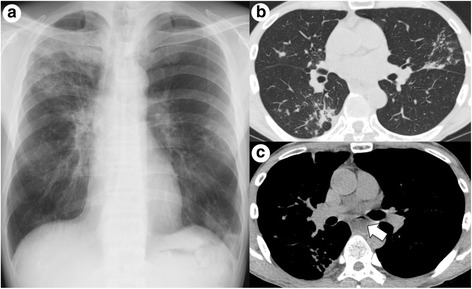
a Chest imaging performed during the referral showed infiltrates in the right upper and left lower lung fields and bilateral middle fields. b Computed tomography images showed consolidations and nodular shadows in multiple lung lobes. c The esophagus was dilated with food residue (arrow)
